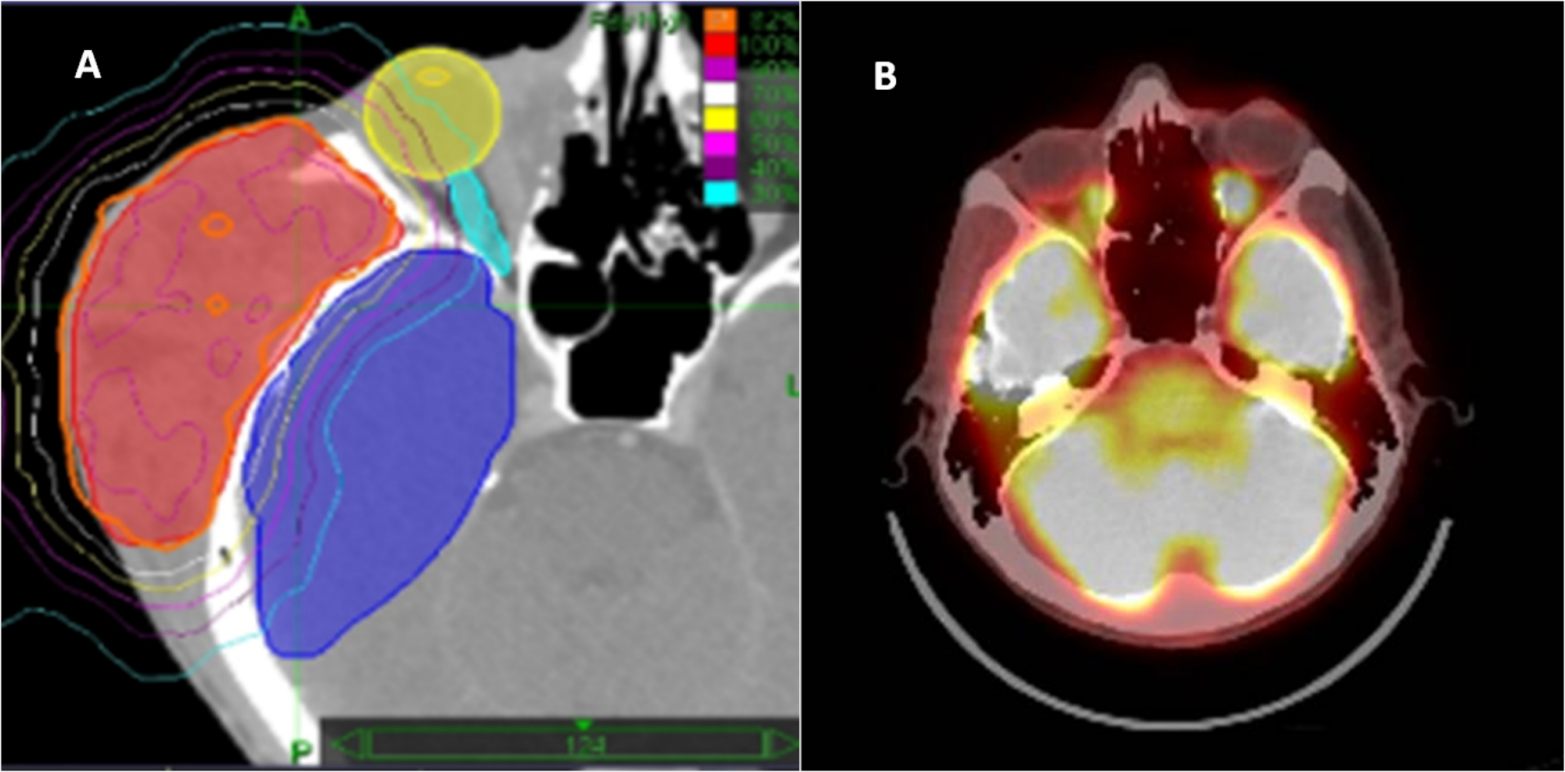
Radiotherapy treatment plan and the response to radiotherapy 2A. The hypofractionated stereotactic radiotherapy (HSRT) plan showing the lesion covered by the 95% isodose line. The dark blue area showing the right temporal lobe, the yellow area showing the right eye, and the light blue area is the right optic nerve. 2B. Positron emission computed tomography performed eight months after HSRT showing a complete response at the irradiated site.
